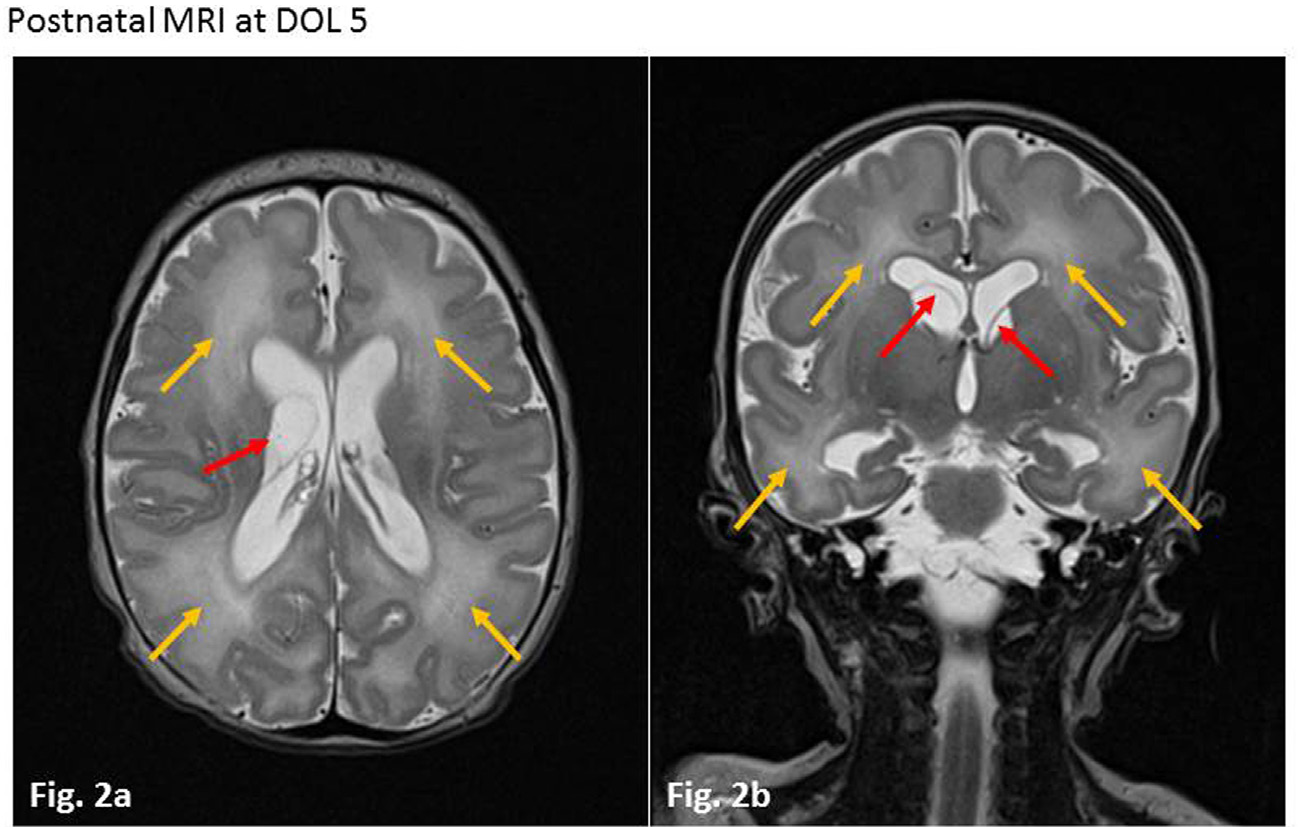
Postnatal magnetic resonance imagings (MRIs) at day of life 5. Axial (A) and coronar (B) T2-weighted sequences. Red arrows show septa of intraventricular cysts in the area of the former matrix germinativa (loco classico). Slight dilatation of both lateral ventricles. Yellow arrows mark white matter hyperintensities bi-frontally and occipitally. No signs of calcification or polymicrogyria.
